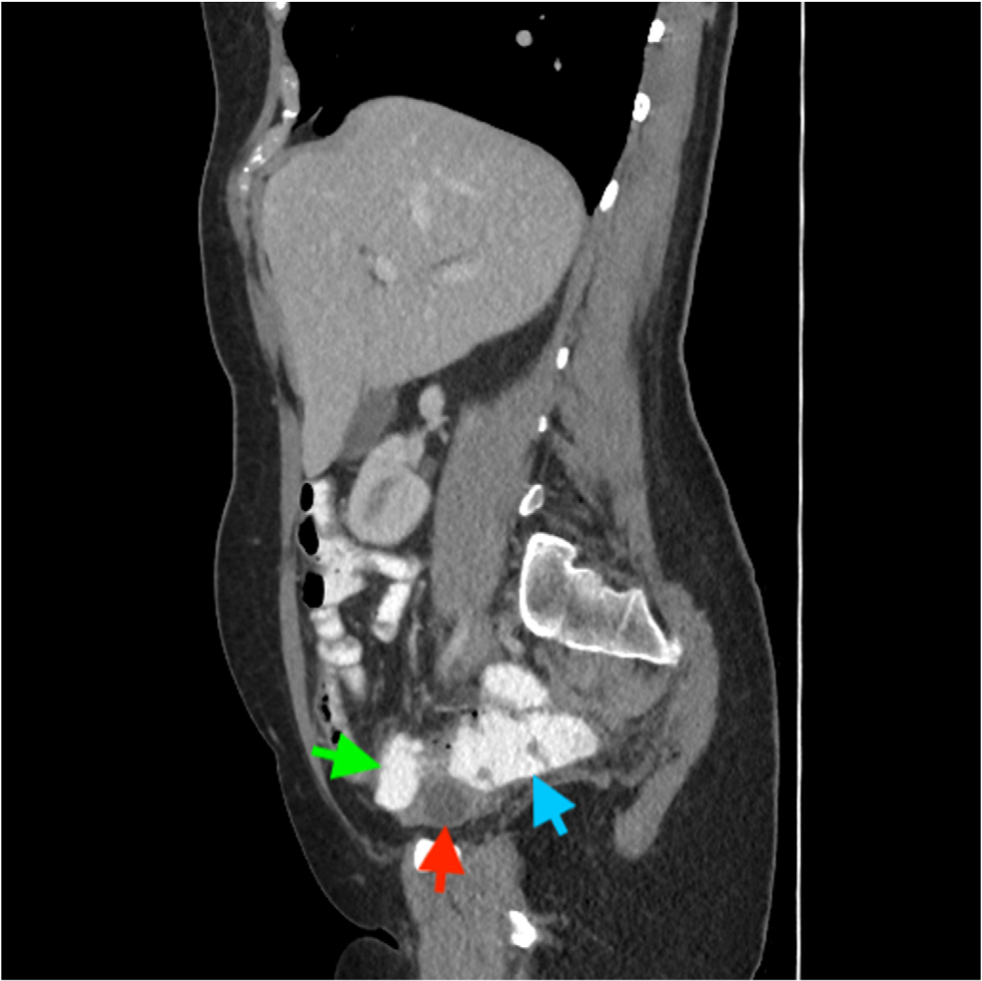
A 16 mm right ovarian dominant follicle (red arrow) seen on computed tomography compressing a low‐lying ascending colon (green arrow), just inferior to a patulous retroverted cecum (blue arrow).
